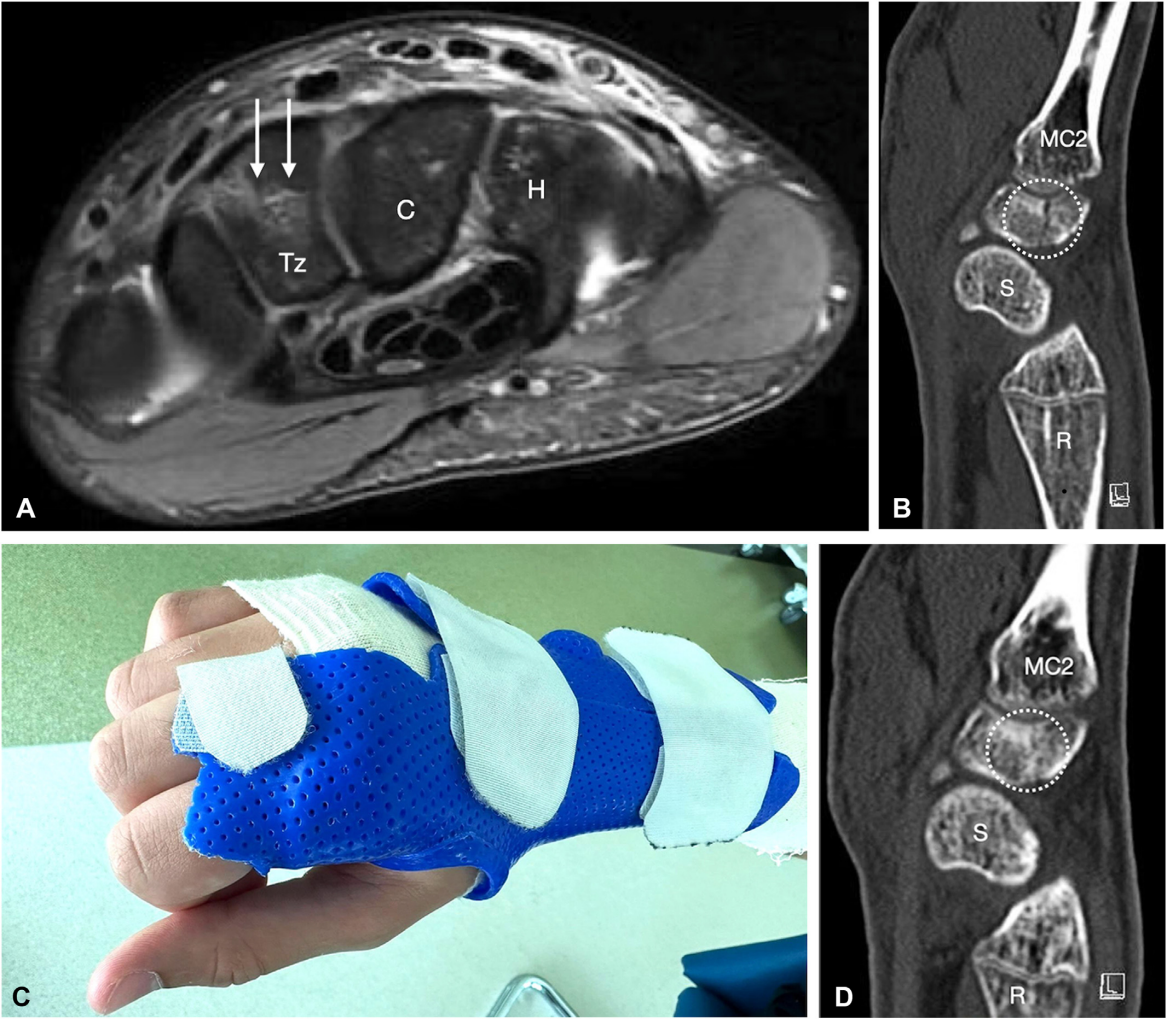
Images of the injury reported in case 1. A MRI T2 axial view showing a complete coronal oblique fracture of the trapezoid with surrounding bone edema (arrows). Tz = Trapezoid; C = capitate; H = hamate. B CT scan sagittal view after 3 weeks with visible trapezoid fracture line and questionable central bone bridging (dotted circle). MC2 = metacarpal 2; S = scaphoid; R = radius. C Thomine splint immobilizing the 2^nd^ and 3^rd^ metacarpophalangeal fractures and the wrist joint. D CT scan sagittal view with complete consolidation of the trapezoid fracture (dotted circle). MC2 = metacarpal 2; S = scaphoid; R = radius.
